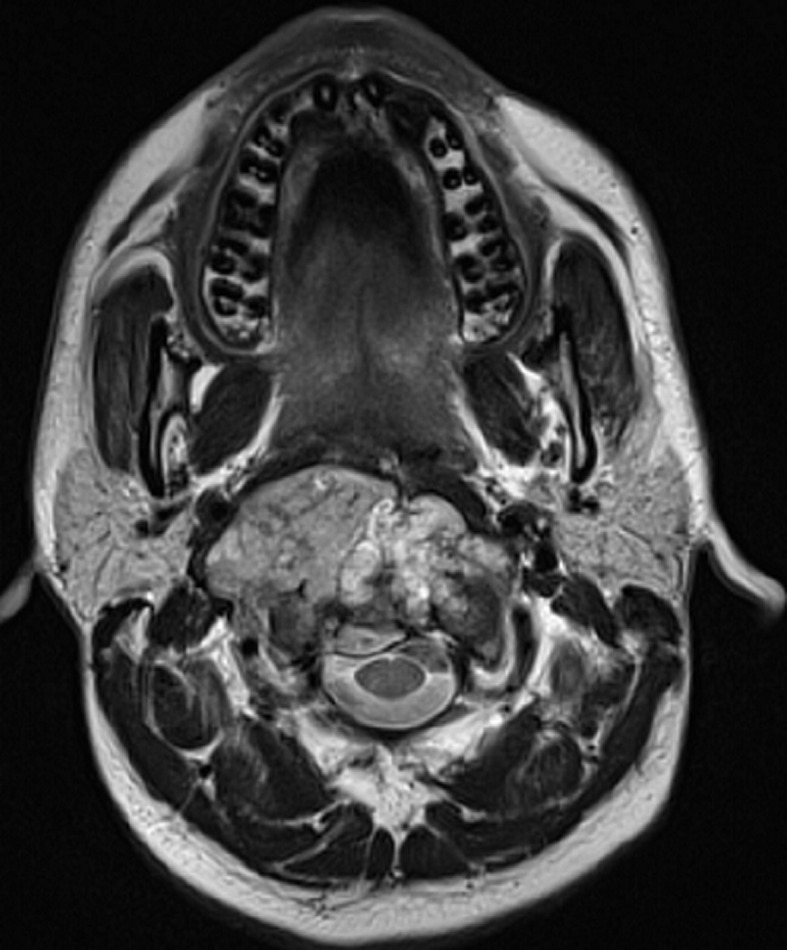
cerebro-spinal MRI, a locally aggressive lesion of the clivus invading the right cavernous sinus (axial cut of brain)
